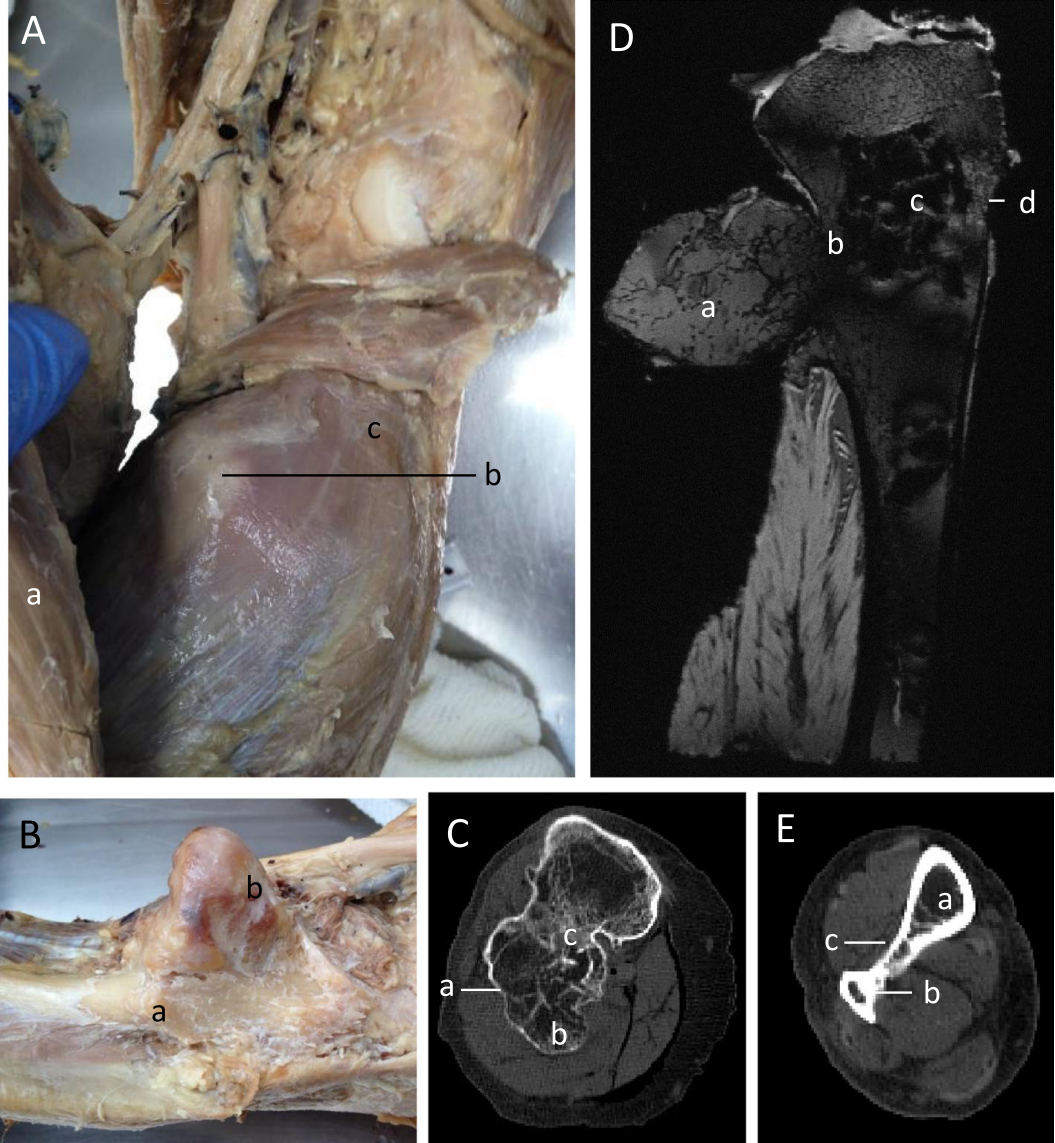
Right proximal leg. a Superficial dissection. Gastrocnemius (a) has been reflected to show the osteochondroma (b) of the head of the fibula causing deformity of the soleus muscle (c). b Lateral view. (a) Fibula. (b) Cartilage capped osteochondromas of head of fibula. c Transverse CT scan through fibular head. Osteochondroma visible with cortical bone (a) and trabecular (b) continuous with the underlying normal bone. A synostosis between the tibia and fibula can be seen (c). d DESS MRI sagittal section. The osteochondroma of the fibular head can be seen containing trabecular bone (a). The synostosis between the fibular and tibia heads can be observed (b). Like the femur and radius the tibia contains large gaps in the trabecular bone structure (c). Cortical destruction has occurred (d). e Transverse CT scan through distal tibia and fibula. An osteochondroma on either the tibia (a) or fibular (b) has formed a synostosis (c) at the distal end of the two bones
